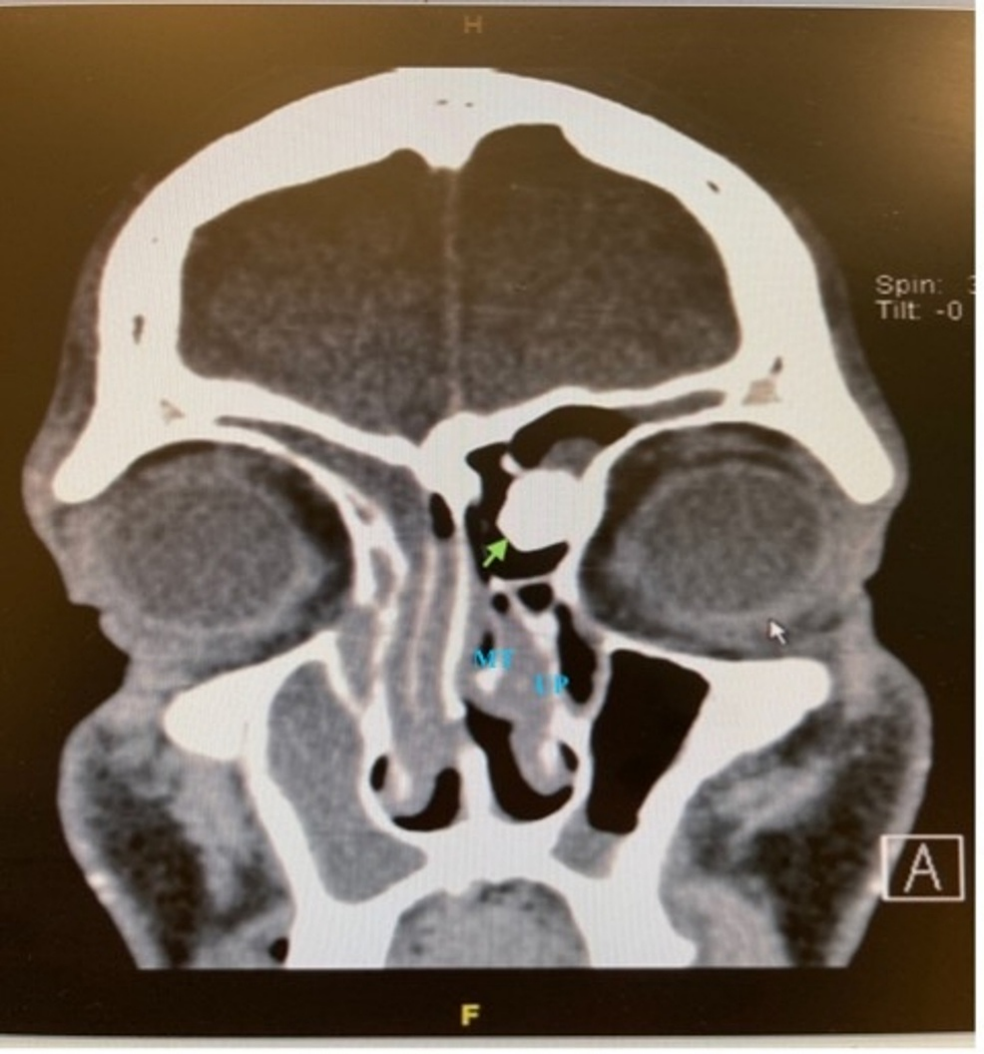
Coronal view of the patient’s CT PNS The osteoma is indicated by the green arrow. MT: middle turbinate; UP: uncinate process; CT: computed tomography; PNS: paranasal sinus
